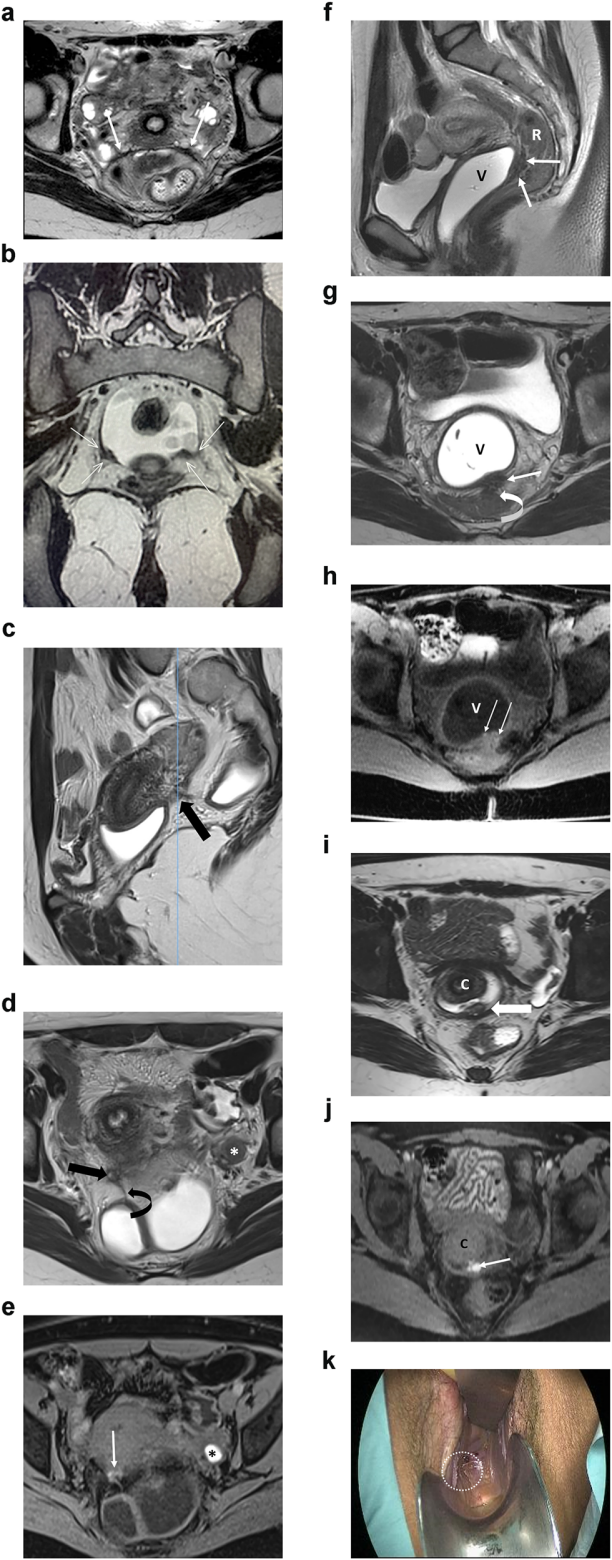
Different imaging examples of endometriosis affecting the torus uterinus and USLs. a Bilateral involvement of the USLs in a 35-year-old woman with dyspareunia and pelvic pain. The axial T2W MR image shows proximal USL thickening with low signal intensity and irregular margins (white arrows), known as an arciform pattern. b Bilateral involvement of the USLs in a 17-year-old woman with severe dysmenorrhea. The coronal T2W image demonstrates asymmetric thickening of the proximal thirds of the USLs (arrows). c–e Endometriosis affecting the right USL in a 27-year-old woman with dysmenorrhea and dyspareunia. The sagittal (c) and axial (d) T2W MR images show nodular thickening of the proximal third of the right USL (arrows). There is also a fibrous septum attached to the adjacent bowel serosa (curved arrow in d) and a small left ovarian endometrioma (* in d). The axial T1WFS MR image (e) depicts hyperintensity foci within both the uterosacral thickening (arrow) and the ovarian endometrioma (*). f–h Endometriosis affecting the rectovaginal septum in a 35-year-old woman with dyspareunia. The sagittal (f) and axial (g) T2W MR images demonstrate nodular thickening with low signal intensity of the rectovaginal septum (arrows), associated with adhesion to the anterior rectal wall (curved arrow in g). The axial T1WFS MR image (h) shows tiny hyperintensity foci within the nodule (arrows). i–k Vaginal endometriosis in a 30-year-old woman with deep dyspareunia. The axial T2W MR image (i) shows nodular thickening occupying the posterior vaginal fornix (arrow). The axial T1WFS MR image (j) depicts hyperintensity foci within the nodule (arrow). Image (k) obtained during vaginal examination demonstrates endometriotic infiltration of the posterior vaginal fornix (white circle). R, rectum; V, vagina; C, cervix
